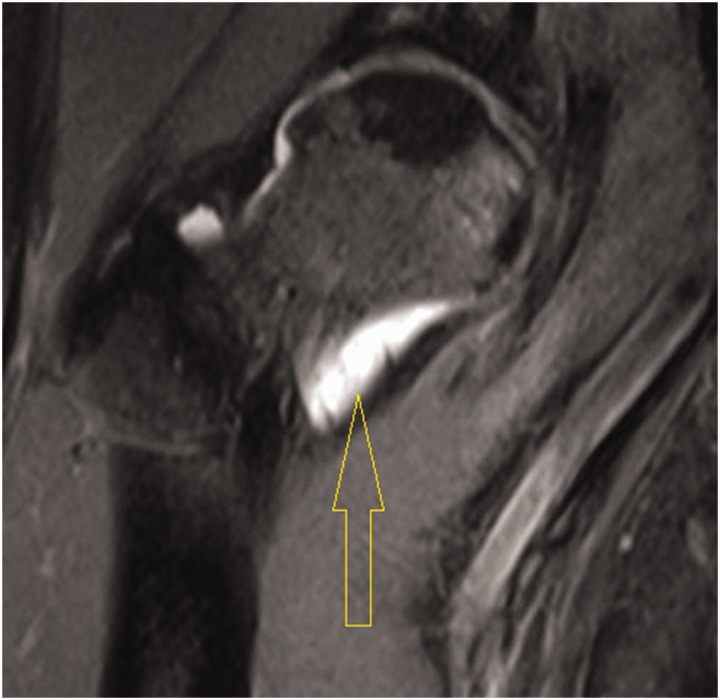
MRI showing significant joint effusion.
